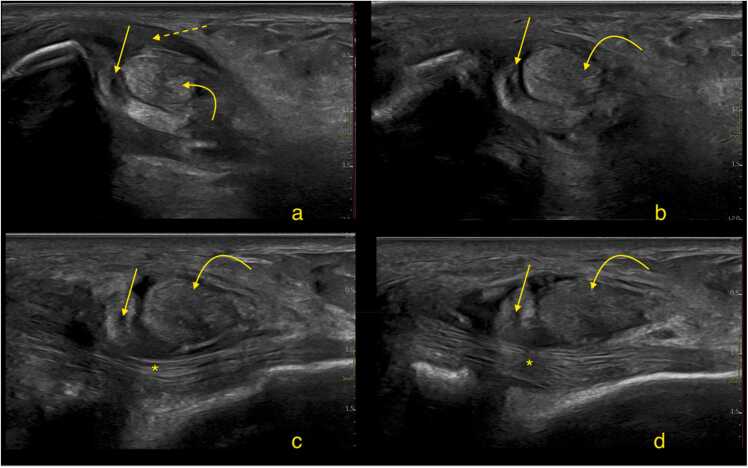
A runner presented with pain in the area posterior to the lateral malleolus. Ultrasound revealed a partial peroneus brevis split tear beginning at the level of the lateral malleolus with ultrasound-*cleft sign*. The tear extends inferiorly relative to the apex of the lateral malleolus (straight arrow). The peroneus longus is marked with a curved solid arrow, shows pathological changes, including swelling and abnormal echogenicity, consistent with advanced tendinopathy. The superior peroneal retinaculum is slightly thickened (dashed arrow). The calcaneofibular ligament is indicated by an asterisk. Cross-section a is the highest, while section d is the lowest. Sections a and b are transverse, while sections c and d are oriented obliquely, perpendicular to the course of the peroneal tendons.
